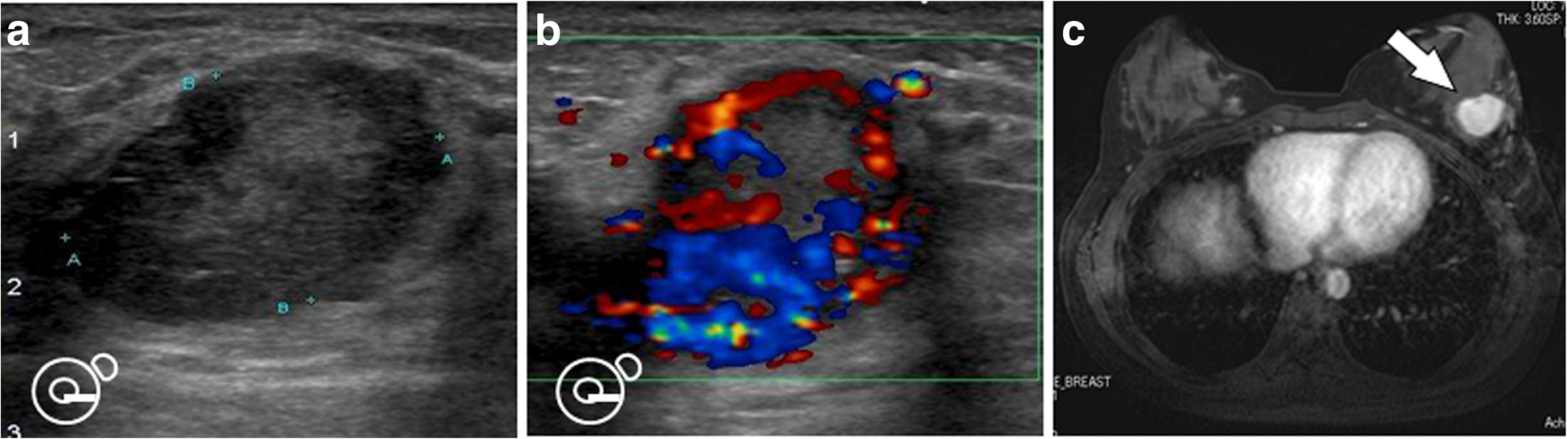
Ultrasonography and MRI image findings: The mammary tumor was revealed as a hypoechoic, internally heterogeneous mass measuring 22.4 × 16.2 × 21.1 mm (a) with a rich blood supply (b) using ultrasonography, while magnetic resonance imaging findings identified a 3-cm tumor larger than that found on prior imaging (c) (arrow)
